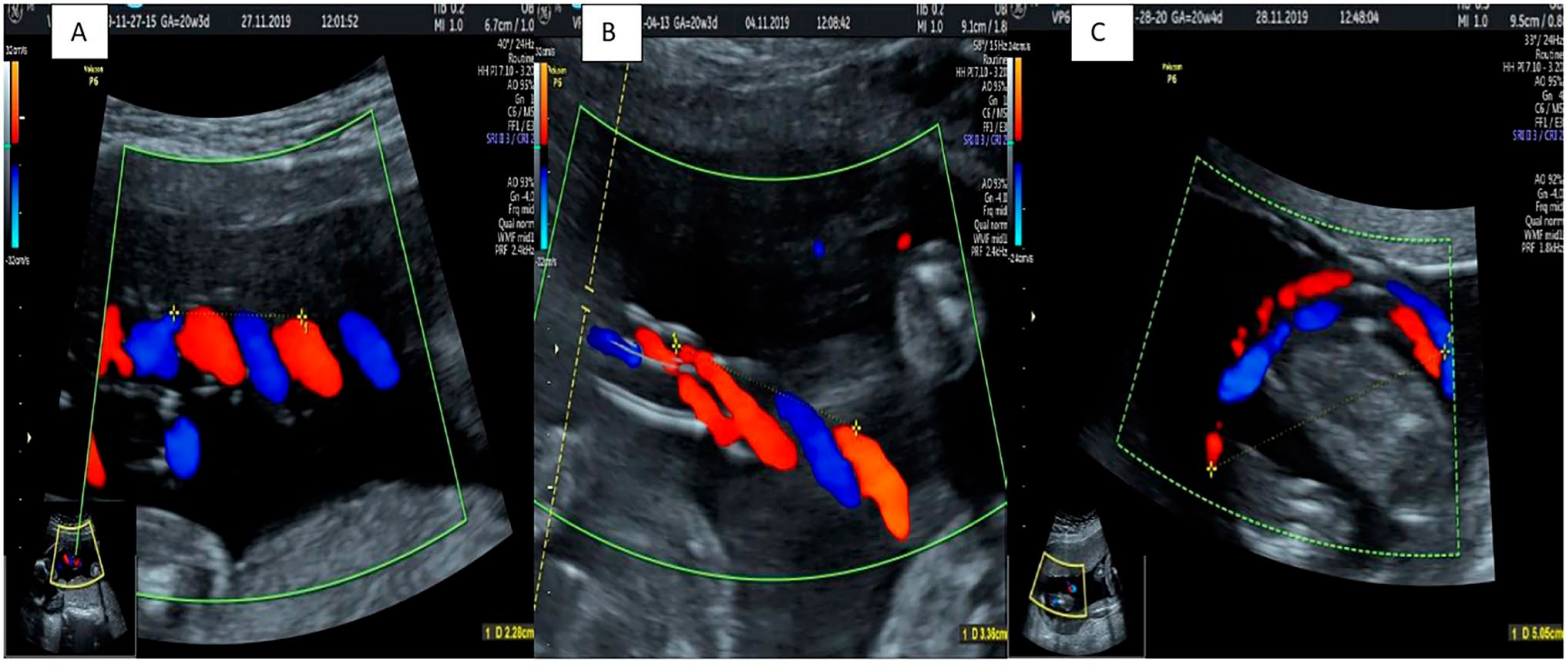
Ultrasonographic assessment of umbilical cord coiling patterns
